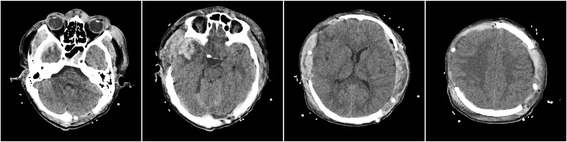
Computed tomography following the third decompressive surgery
